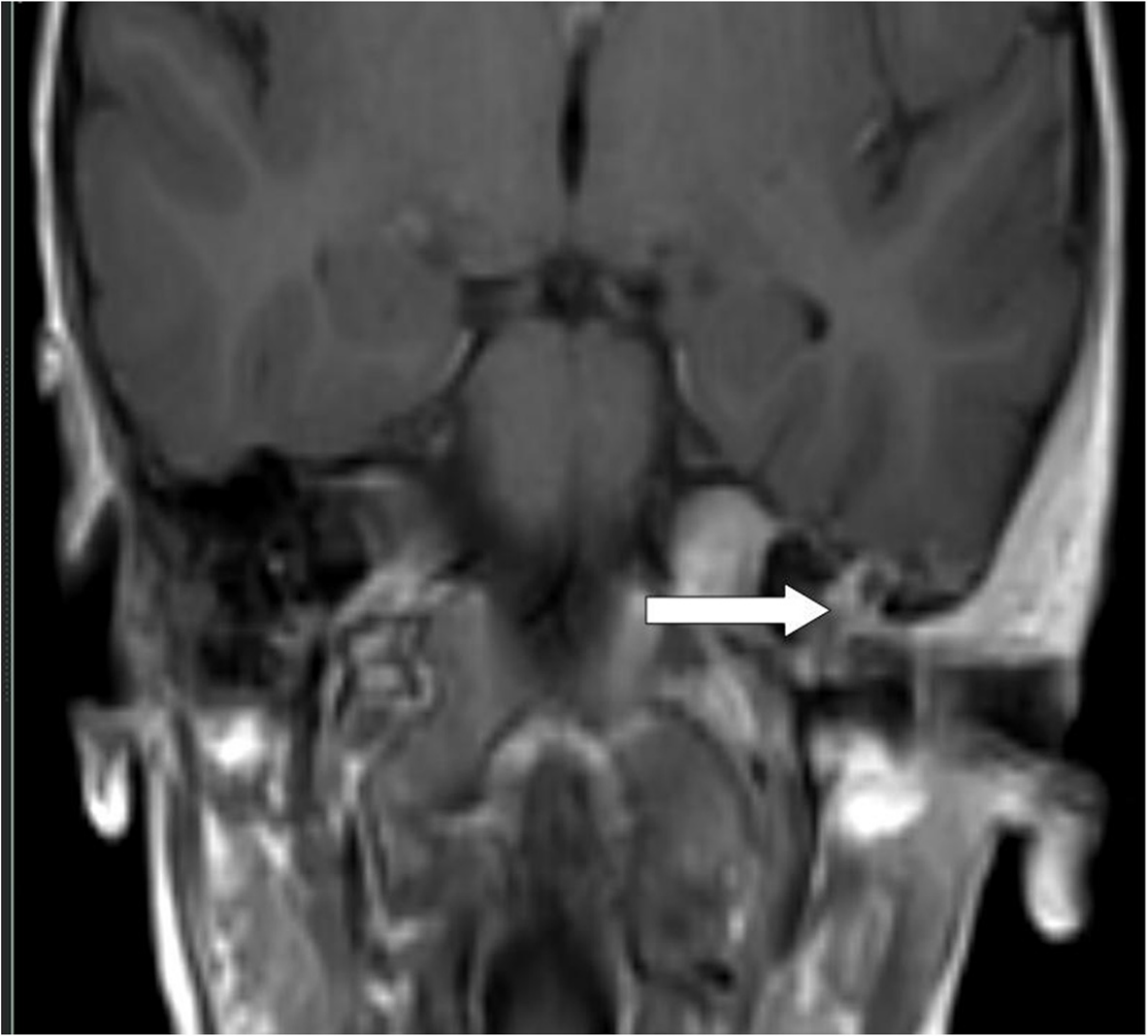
MRI coronal section T1 + Gadolinium. Arrow shows area with enhancement in left pars petrosa.
